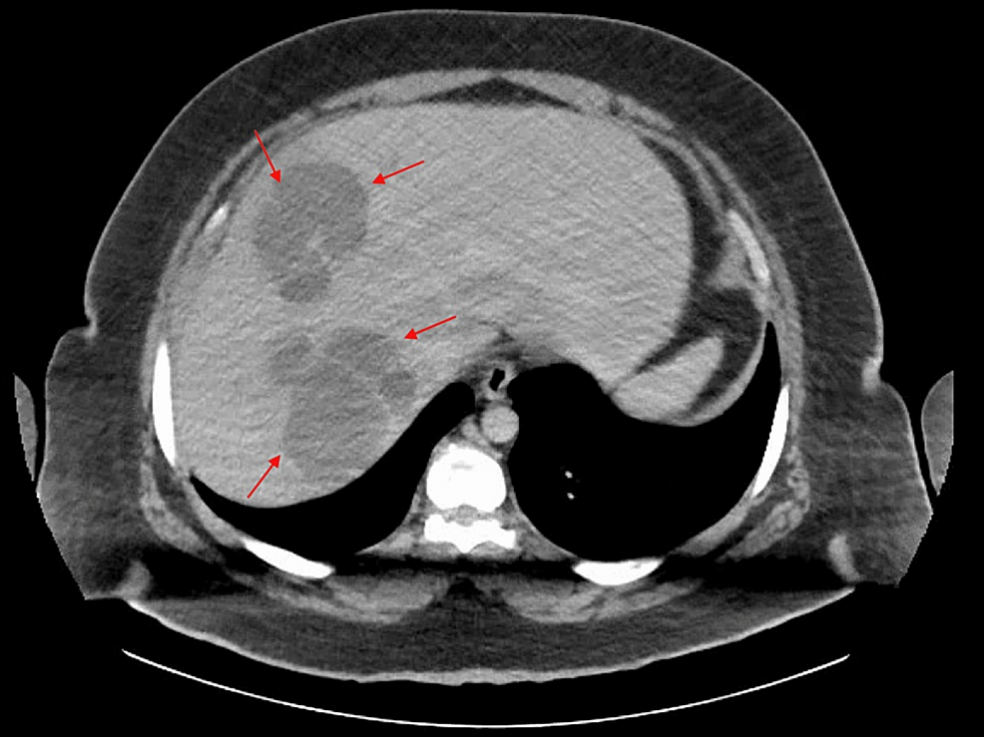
CT abdomen revealing liver lesions Red arrows point to multiloculated liver lesions
